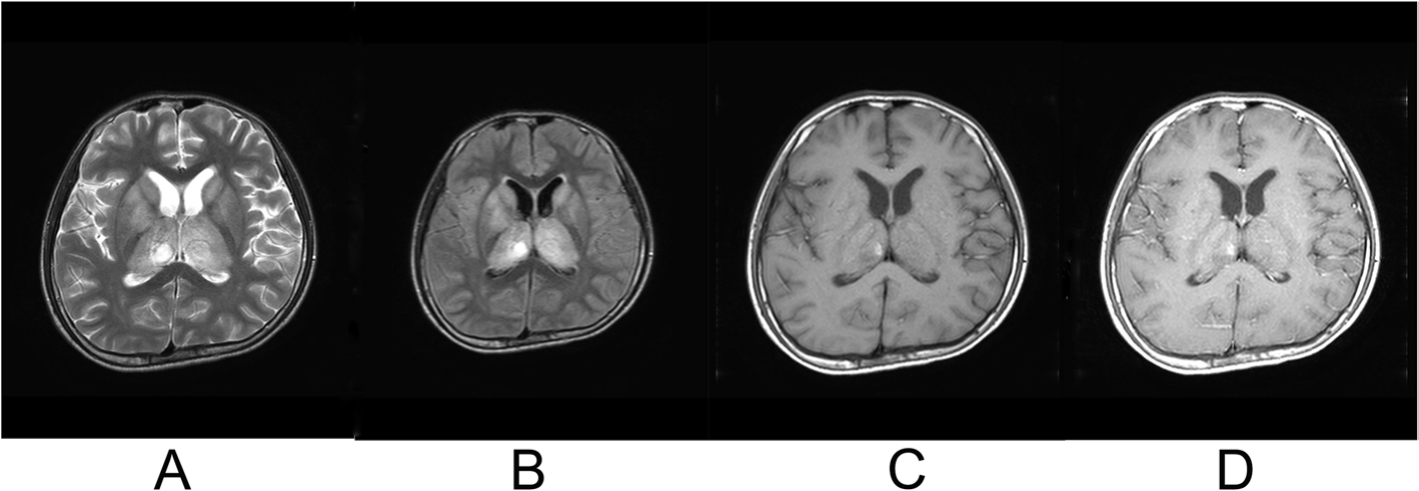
Brain MRI of a 13-year-old boy with acute necrotizing encephalopathy. A Axial view of T2-weighted image (T2WI) show swelling and hyperintense signals (arrow) in both thalami; (B) Axial view of T2 fluid-attenuated inversion recovery sequence (T2-FLAIR) show swelling and hyperintense signals (arrow) in both thalami; (C) equal or slightly low signals on T1-weighted image (T1WI) with internal hemorrhage (arrow) and necrosis (star); (D) contrast-enhanced T1WI shows no enhancing brain lesions
